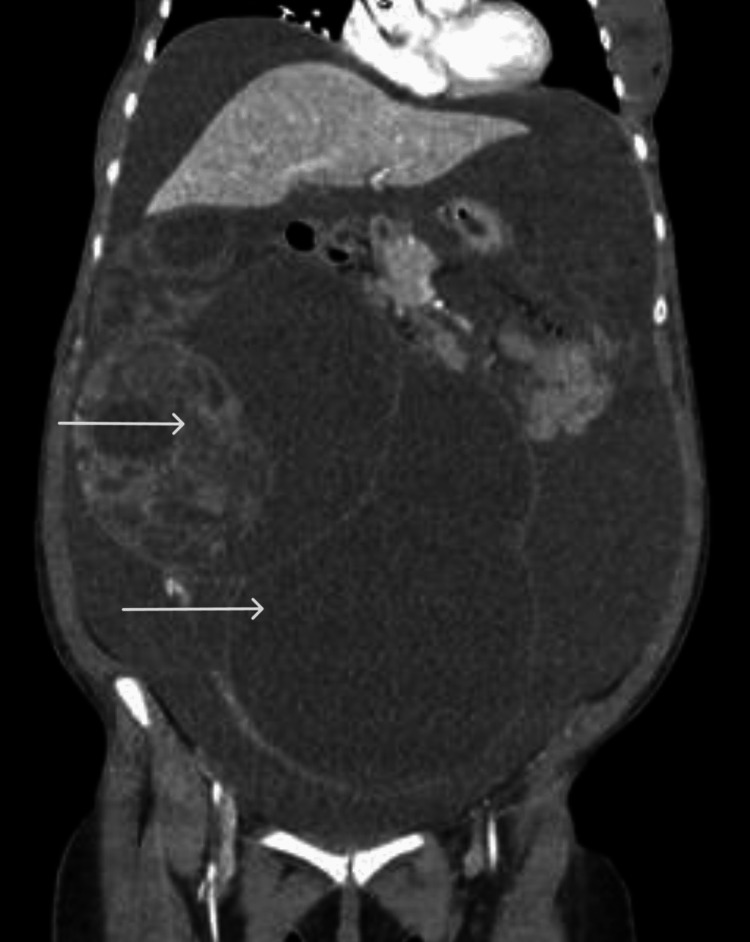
CT of the abdomen and pelvis revealed a cystic mass of the right adnexa
